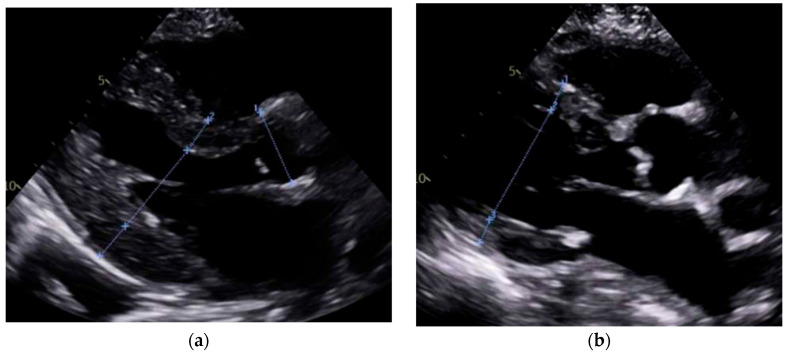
Parasternal long-axis transthoracic echocardiography (TTE) comparison. (a) Cardiac amyloidosis showing diffuse concentric left ventricular wall thickening and sparkling myocardial texture, with a small left ventricular cavity and biatrial enlargement. (b) Cardiac sarcoidosis showing normal to mildly thickened walls, with possible basal septal thinning or regional motion abnormalities, and preserved left ventricular cavity size.
